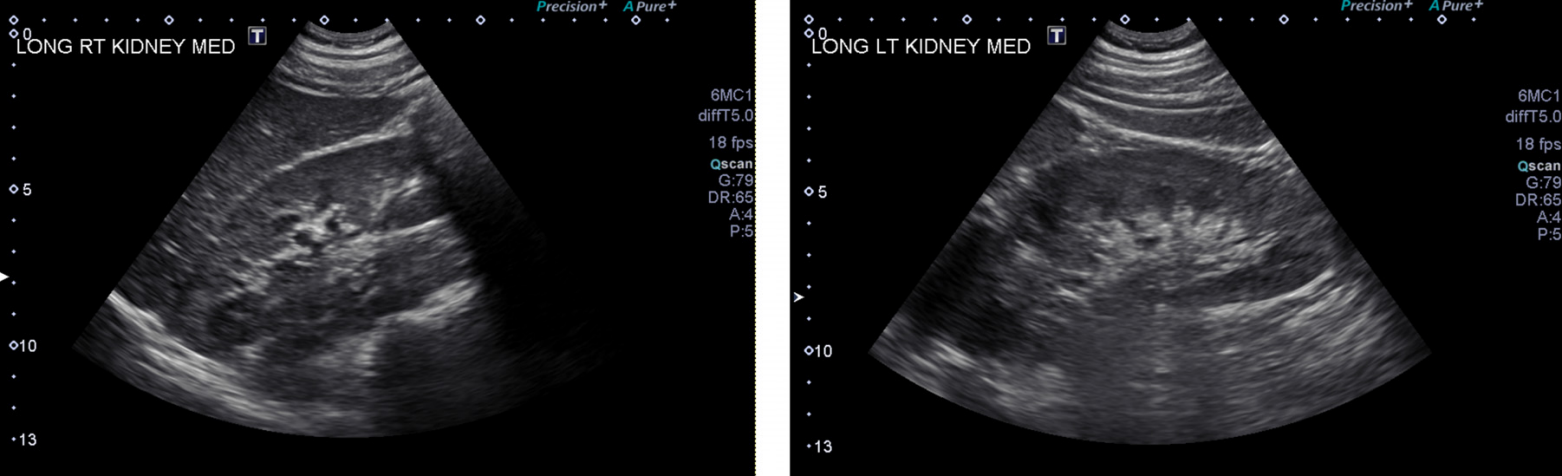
Normal sonogram of bilateral kidneys.
